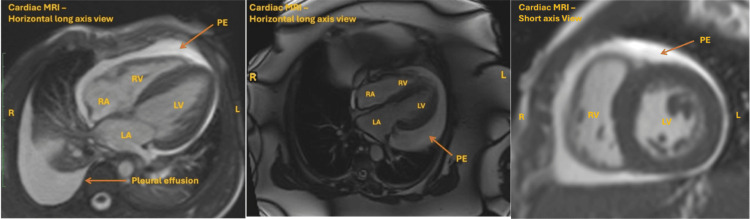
Different views of cardiac MRI demonstrating pericardial effusion. Effusion marked by the orange arrow. (A & B) Horizontal long axis view. (C) Short axis view MRI: magnetic resonance imaging, PE: pericardial effusions, LV: left ventricle, RV: right ventricle, LA: left atrium, RA: right atrium This image has not been published elsewhere.
